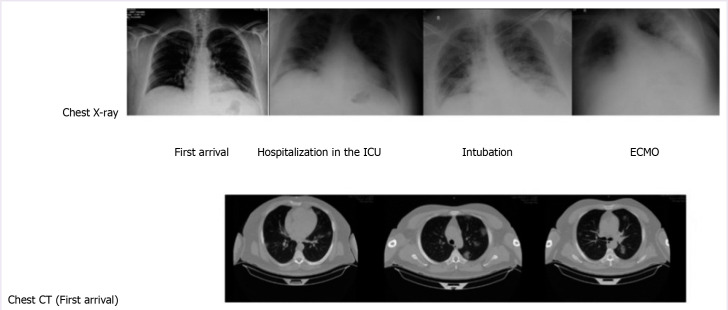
Chest X-ray and computed tomography images of a 48-year-old man with COVID-19 infection.
